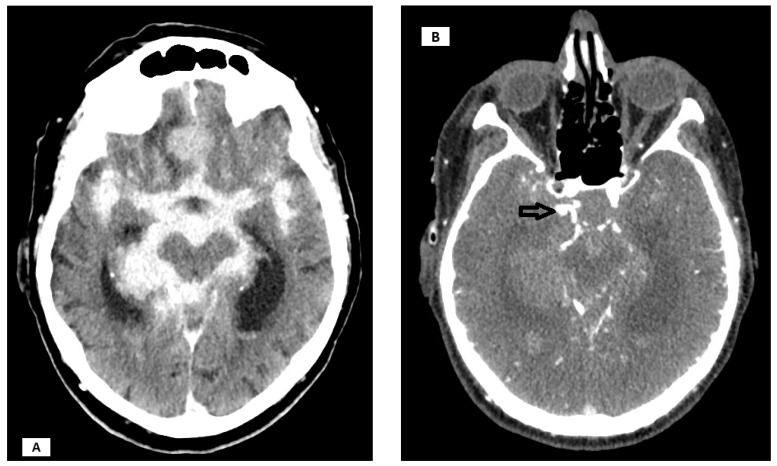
An 86-year-old female patient was admitted with an aneurysmal subarachnoid hemorrhage caused by rupture of an aneurysm located at the C7 segment of the internal carotid artery (as indicated by arrow). Cranial CT incidentally demonstrated a meningioma of the planum sphenoidale (A,B). The aneurysm was treated by microsurgical clipping, and in the same operative session, gross total resection of the meningioma was performed.
